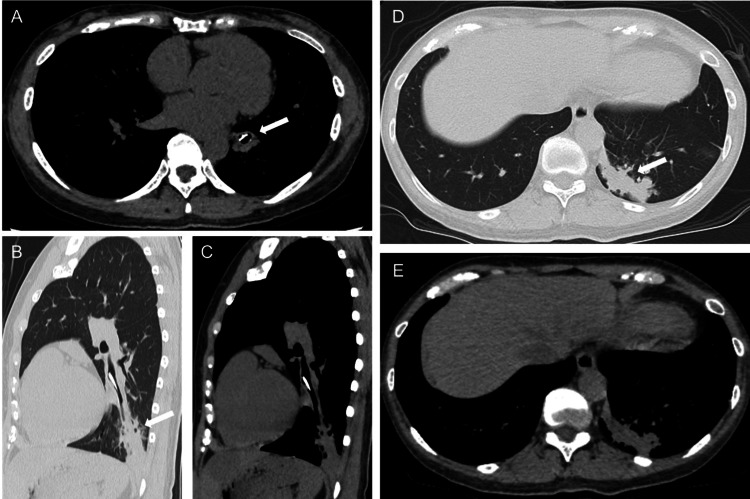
Initial chest computed tomography scan (A) A highly absorbent foreign body is identified at the left inferior lobar bronchus (arrow). (B) Infiltrative opacities extending from the foreign body to the peripheral region are observed (arrow). (C) No high-attenuation mucus is observed within the infiltrative opacities extending peripherally from the foreign body. (D) Club-shaped opacities suggestive of mucus plugs are observed (arrow). (E) No high-attenuation mucus is observed within the club-shaped opacities, suggestive of mucus plugs.
